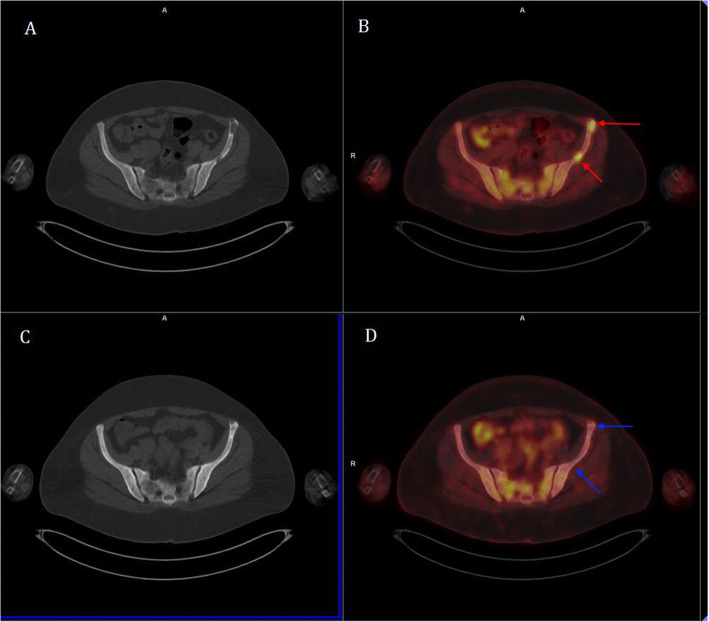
Preoperative and postoperative axial CT and fused ^18^F-FDG PET/CT images: A, B (preoperative) images show hypermetabolic left iliac lytic bone lesions (red arrows) that show complete metabolic resolution in the postoperative ^18^F-FDG PET/CT images (C, D) (blue arrows)
